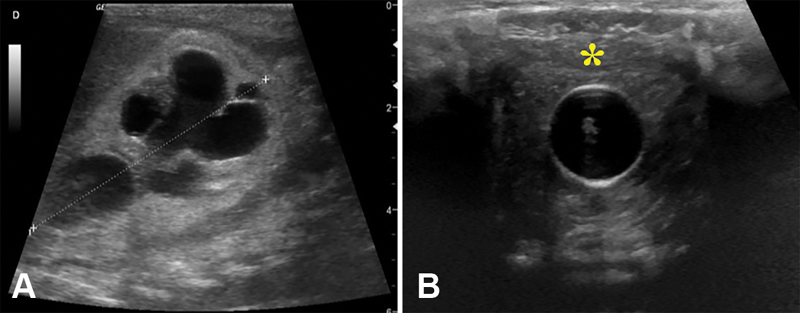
Ultrasound scan showing bilateral ureterohydronephrosis (A) and an empty bladder with the hypertrophied wall (asterisk) closely clasping the balloon of the vesical catheter (B).
